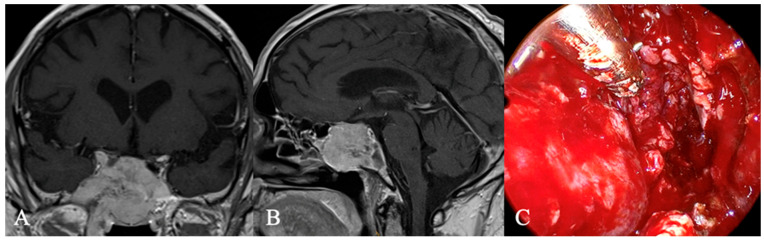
High-grade osteosarcoma. A 75-year-old male patient with high-grade osteosarcoma. (A) Coronal and (B) sagittal contrast-enhanced MRI demonstrate an extensive contrast-enhancing mass with destructive growth pattern along the skull base with the complete pituitary gland and infundibulum encasement. (C) Intraoperative findings revealed firm, well-vascularized tissue infiltrating surrounding structures without clear borders, making gross total resection challenging due to skull base involvement. Postoperatively, he received 50 Gy of radiotherapy and chemotherapy with doxorubicin and gemcitabine.
